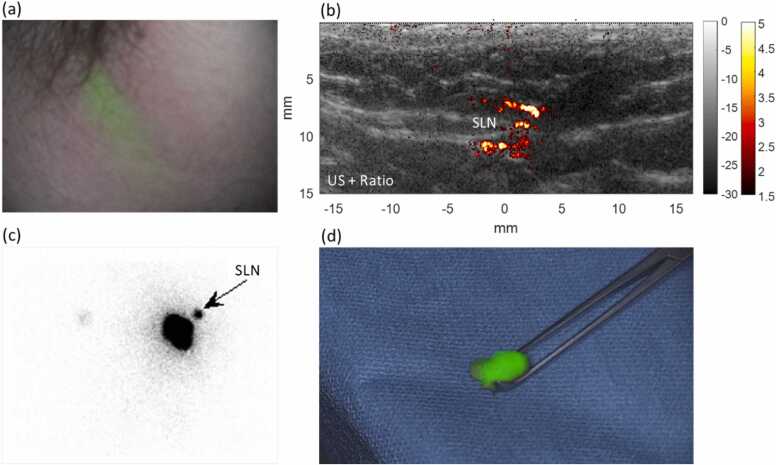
Imaging results from patient No. 5. (a) shows near-infrared fluorescence (NIRF) imaging of a lymphatic vessel indicating a sentinel lymph node (SLN) is located in the axillary basin. (b) photoacoustic ratio image overlaid on ultrasound, showing the ability to locate the SLN at a depth of approximately 10 mm, which the NIRF camera could not. The findings were confirmed with scintigraphy (c) and with intraoperative NIRF (d), confirming the PAI findings.
